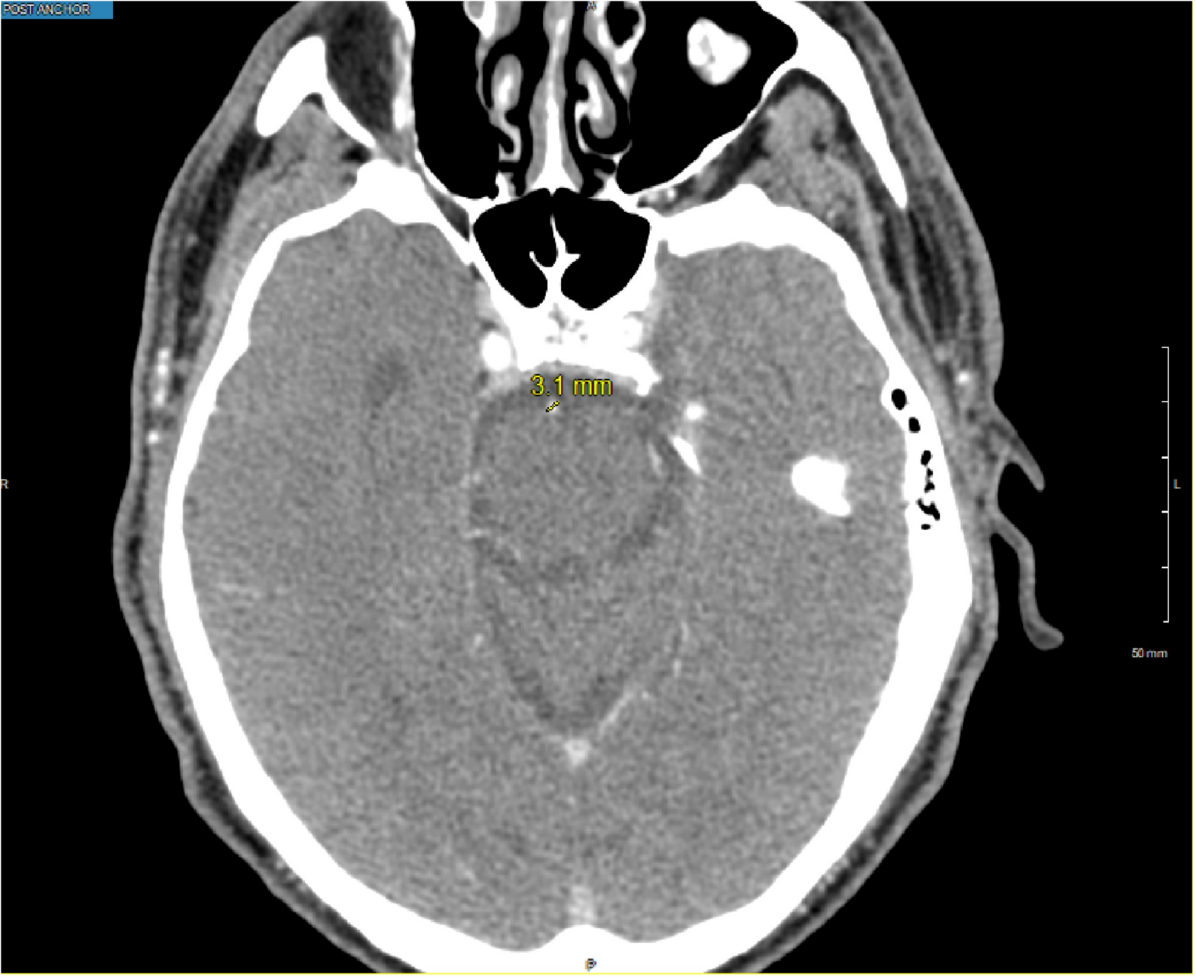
CT angiography (CTA) head performed 48 hours after initiation of calcium channel blocker therapy showing dilation of basilar artery to 3.1 mm.
